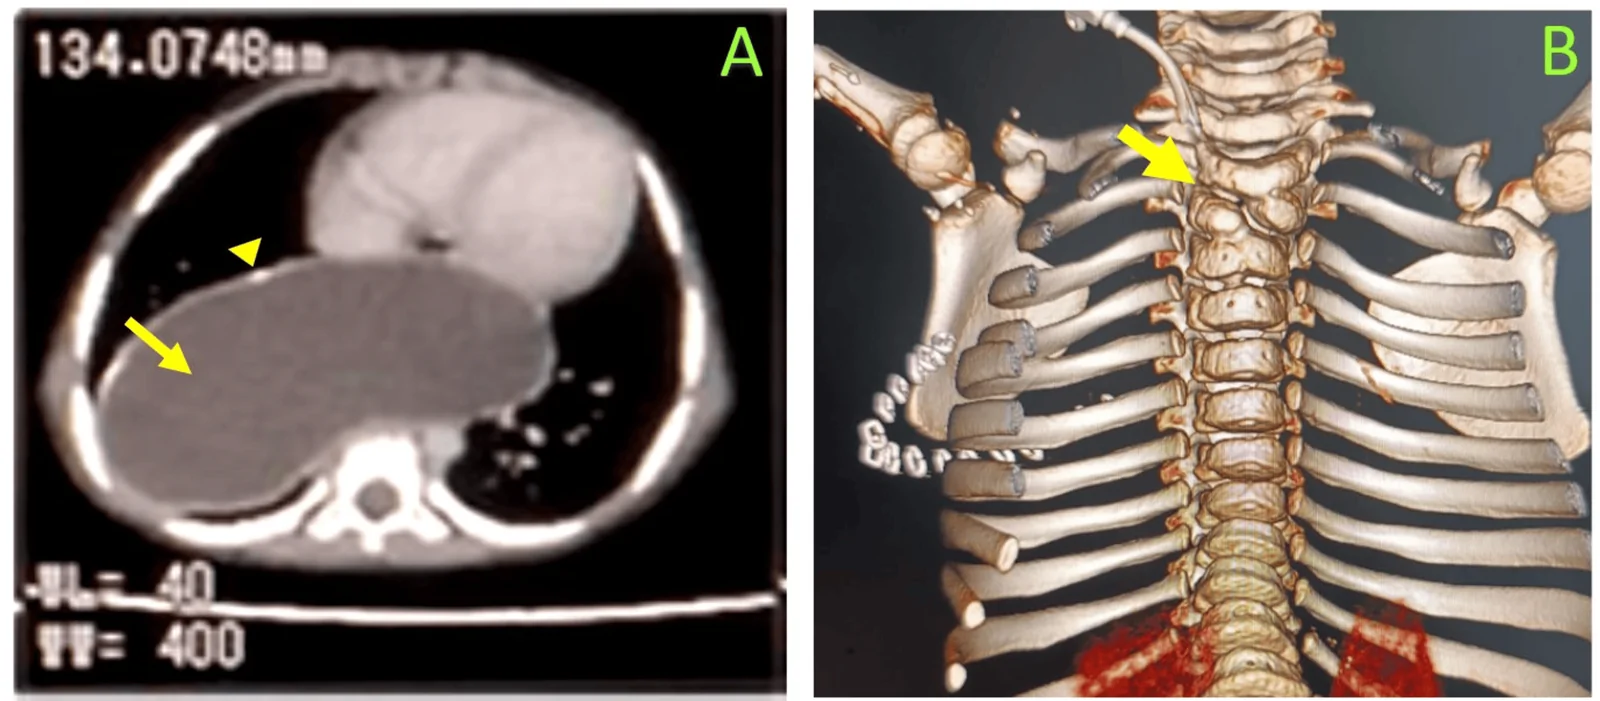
Contrast-enhanced CT and three-dimensional reconstruction. (A) Axial contrast-enhanced CT demonstrating a large posterior mediastinal cystic lesion (arrow) with peripheral rim calcification (arrowhead), extending into the prevertebral region and displacing the soft tissue structures (B) Three-dimensional CT reconstruction demonstrating congenital vertebral anomalies involving the T1 and T2 vertebrae (arrow).
